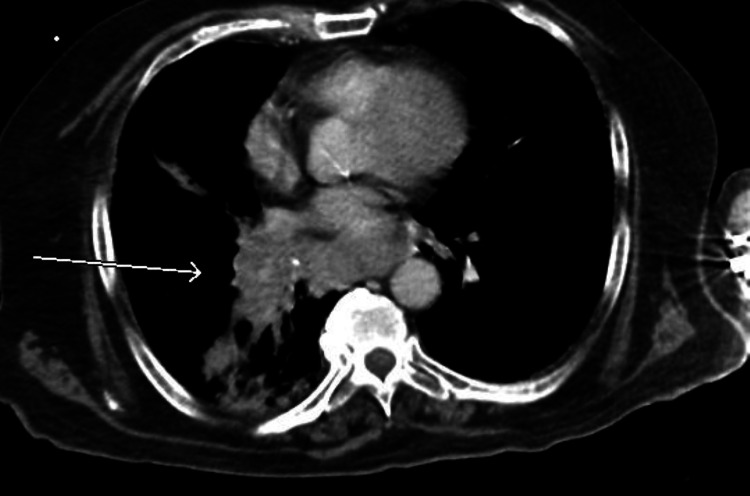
CT of the chest (axial view) showing a mediastinal mass
